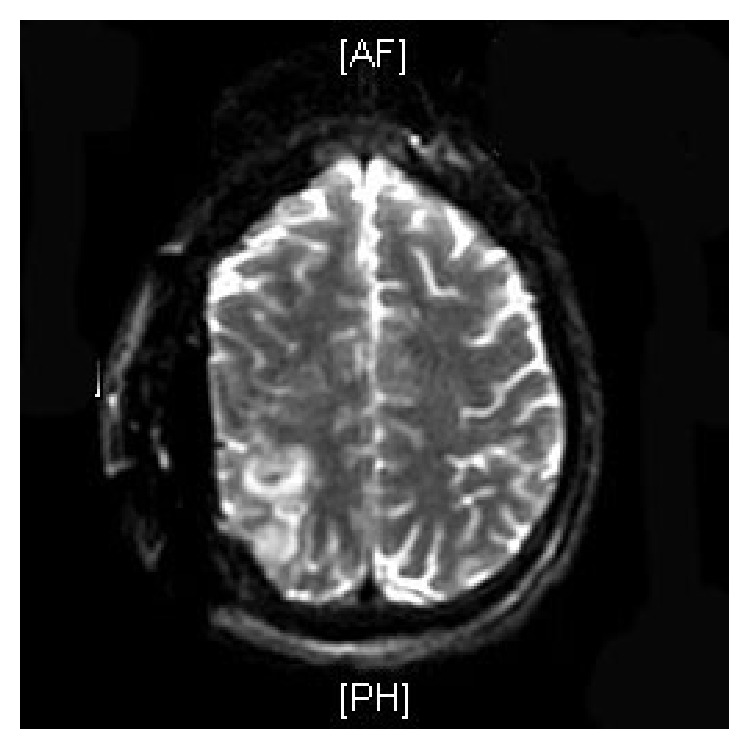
Diffusion weighted magnetic resonance image demonstrating right parietal infarct.
